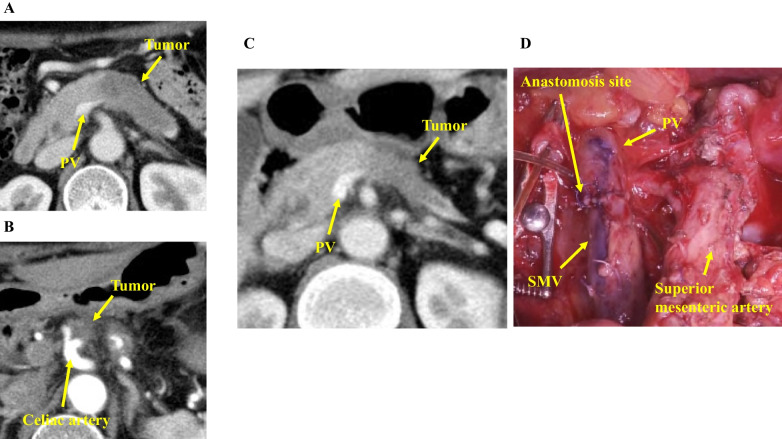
CT before the surgery. A CT before neoadjuvant chemotherapy showing the tumor infiltrating the pancreas. B On CT before neoadjuvant chemotherapy, the tumor was close to the celiac artery. C CT after chemotherapy showing a decrease in the tumor size; however, the tumor is close to the PV. D We resected the PV, including the SV–SMV confluence. *CT* Computed tomography, *PV* Portal vein. *SV* Splenic vein, *SMV* Superior mesenteric vein
